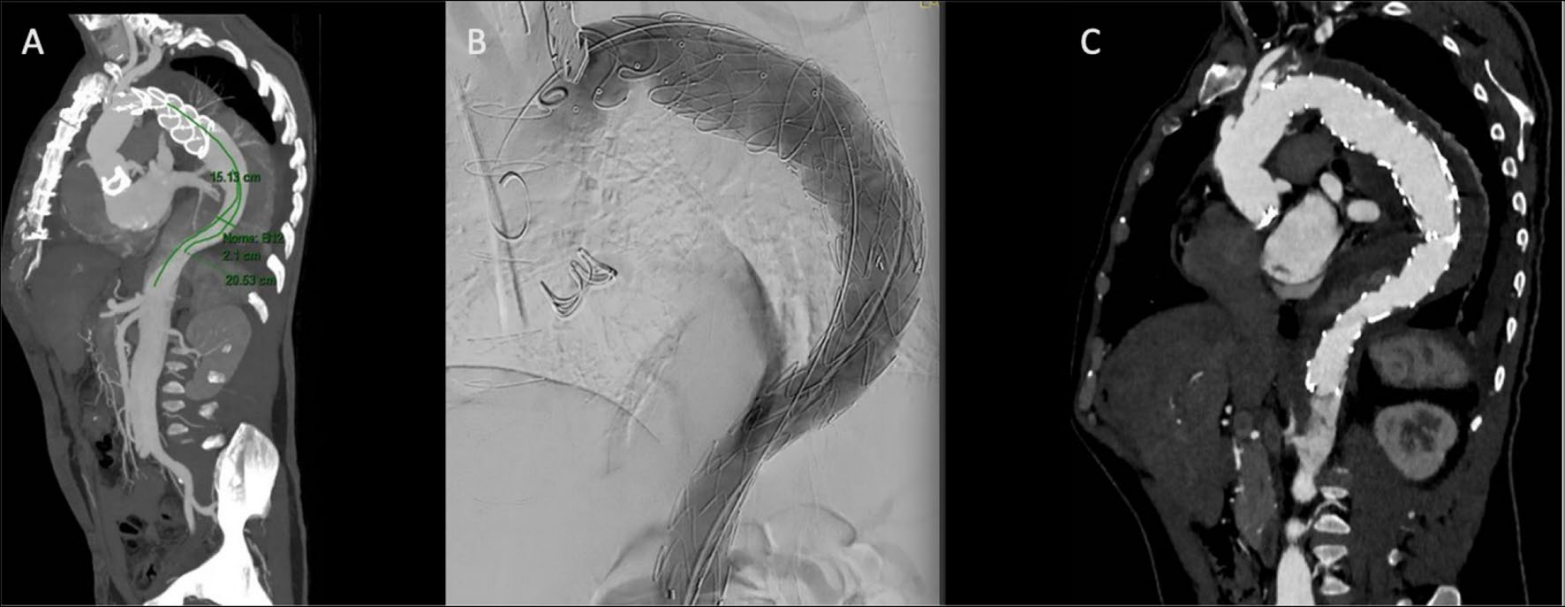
TEVAR extension after FET for residual dissection. A: Preoperative CT scan and planning for endovascular extension. B Angiographic control after TEVAR. C Postoperative CT scan*TEVAR, thoracic endovascular aortic repair; FET, frozen elephant trunk; CT, computed tomography*
